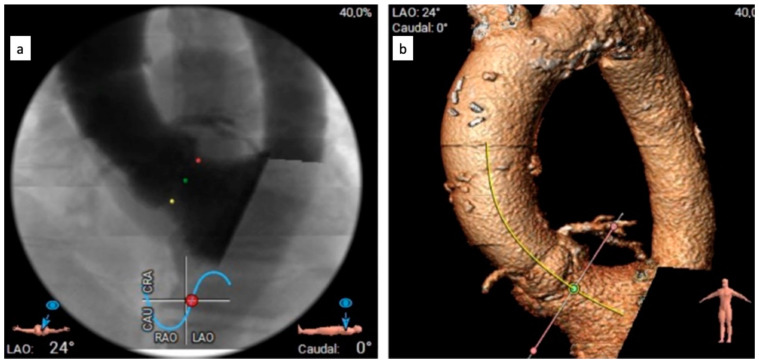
Investigative images: Aortography—aortic root and ascending aorta, and origins of coronary arteries (a) and CTA 3D reconstruction—aortic root with coronary arteries origin, ascending aorta (b).
